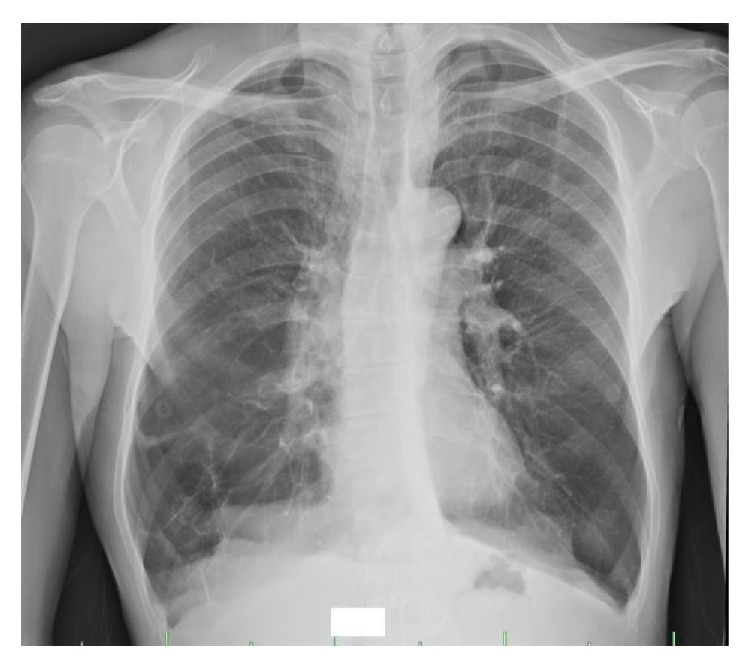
Posttreatment chest X-ray.
